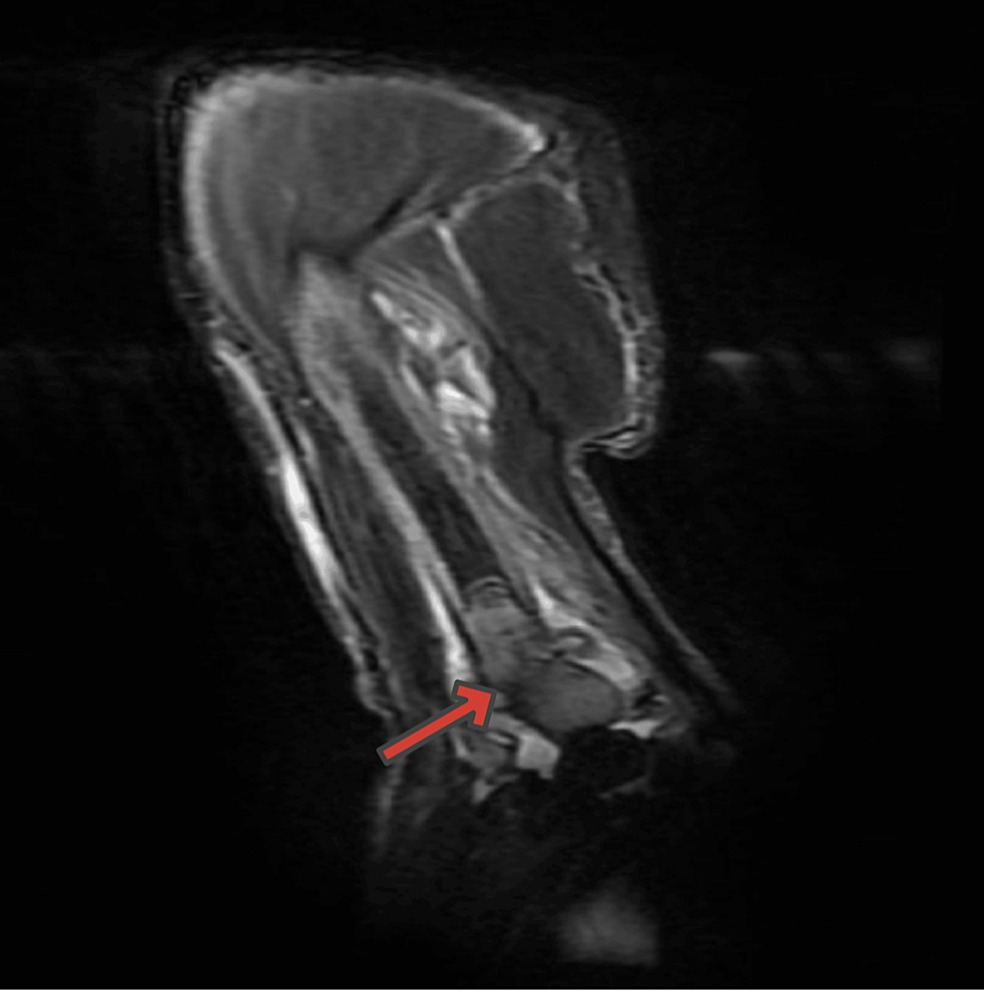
Right humerus MRI: complete destruction of the distal 7 cm of the right humerus manifested by enhancing low T1 signal intensity with cortical destruction and associated soft tissue component (6 × 5.5 cm) (arrow) MRI: magnetic resonance imaging
